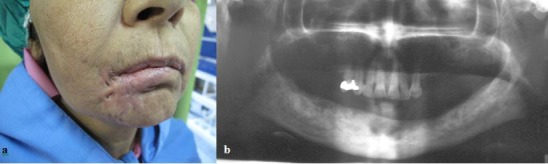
a: Shows scars in the mouth corners of mouth due to herpes zoster  b:  Panoramic radiograph showing a well demarcated sequestrum in the mandibule.
